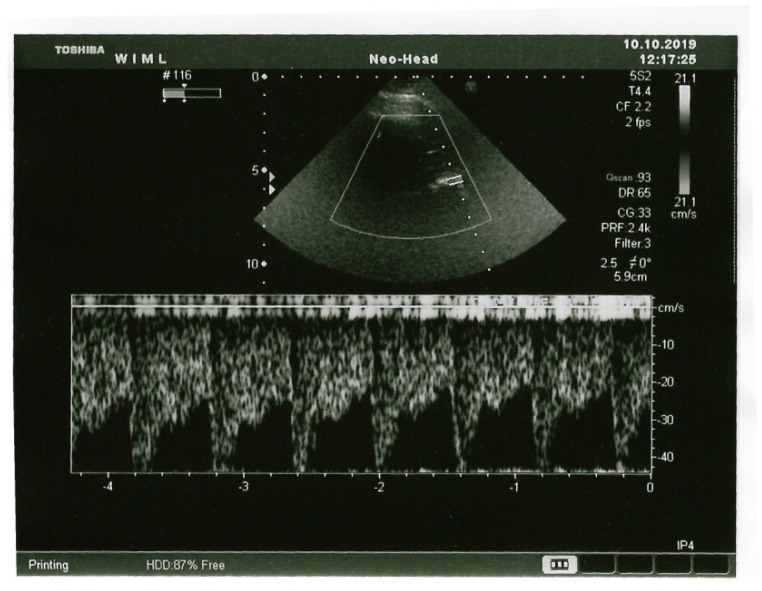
Ultrasound recording of the MCA at the M1/M2 level (M. Abakumow, own collection).
